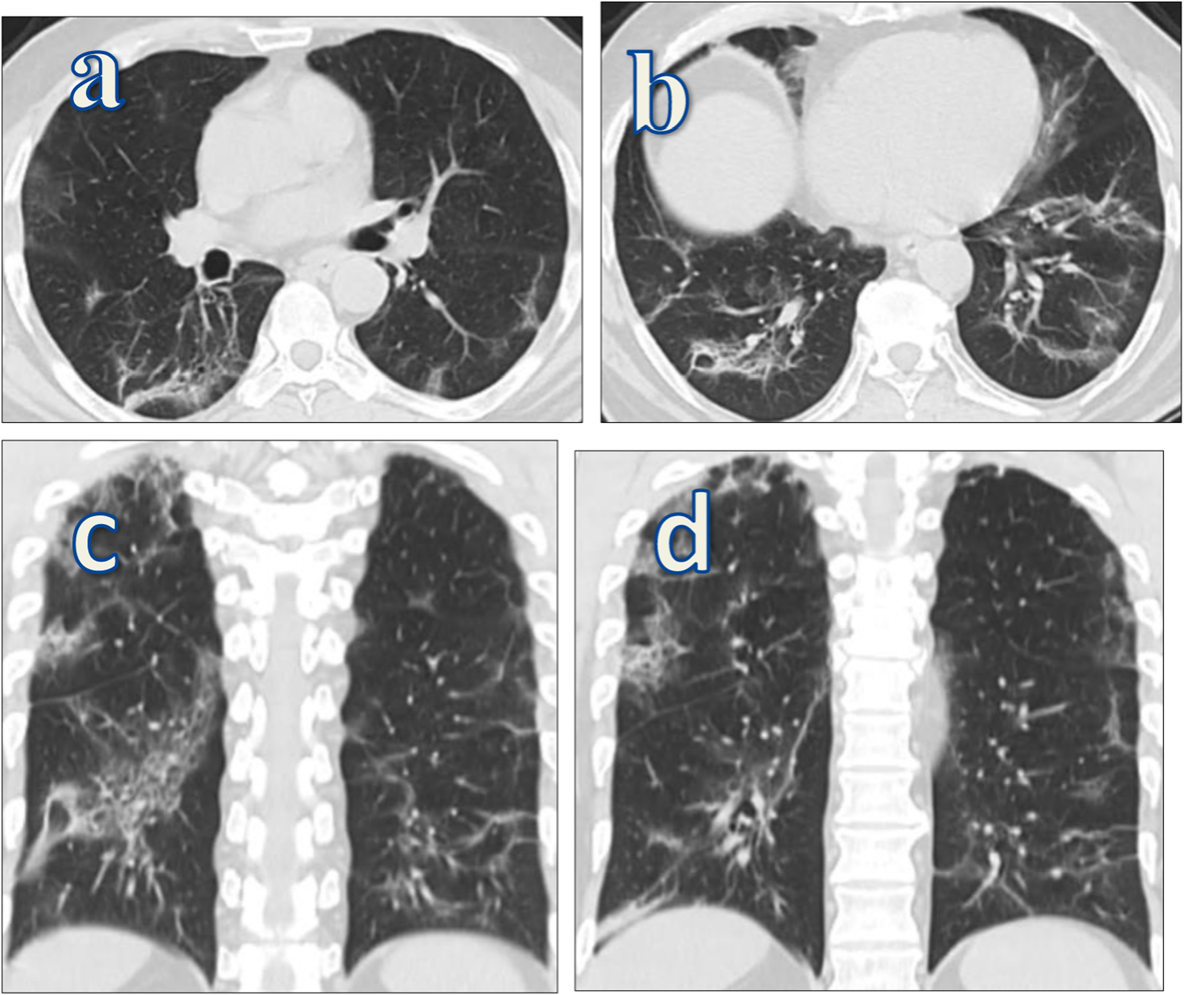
A 56-year-old cigarette smoker male patient presented with ferver, dyspnea, and dry cough diagnosed as positive for COVID-19 by PCR. CT-SS on admission was 16/25. Follow-up axial (a,b) and coronal (c,d) MSCT chest was done after 6 weeks from start of symptoms revealed bilateral pulmonary fibrotic changes in the form of fibrotic bands, peribronchial thickening, traction bronchiactasis. and bronchioloctasis
